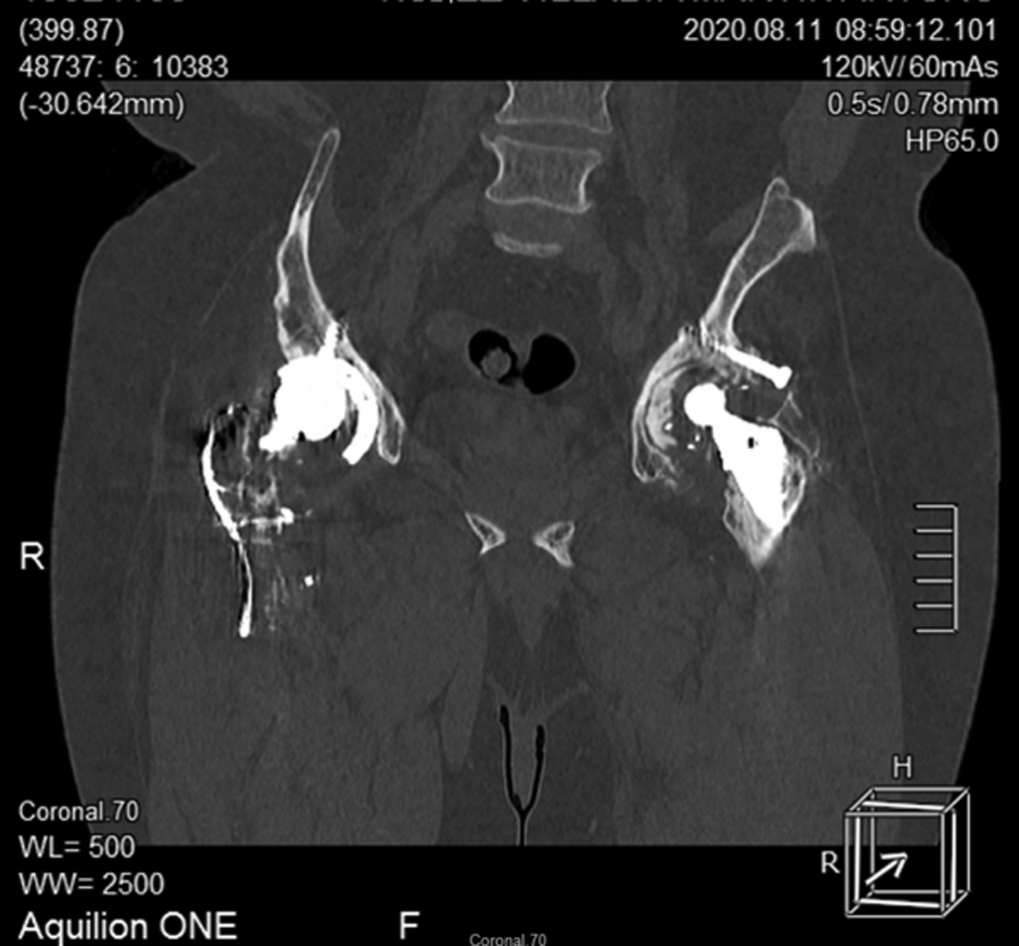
Coronal computed tomography image showing eccentricity of the metal head. Anteversion was reported to be 25°, and inclination was measured at 48°.
